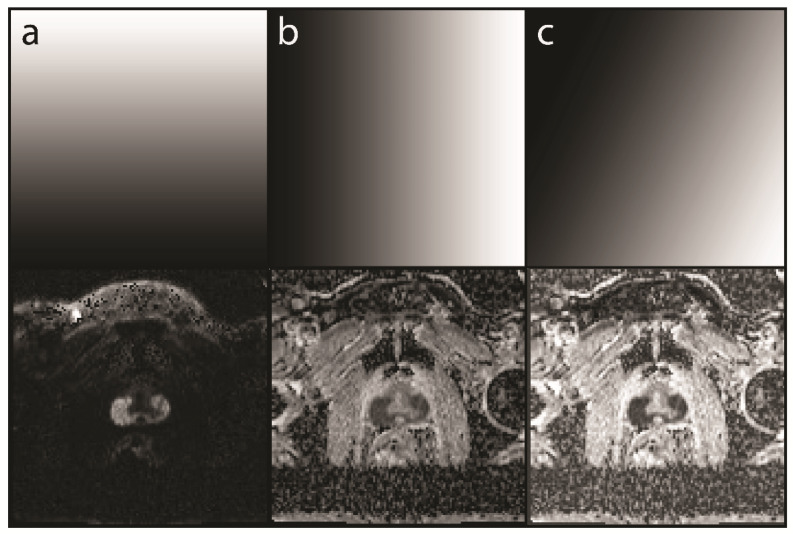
Examples of different readouts from a mcDI dataset created from a high b-value DWI (hbDWI), the ADC, and an intensity map. The selected map is shown in the top row and the resulting mcDI in the bottom row. (a) A readout with a strict vertical gradient yields the original DWI. The resulting image contains no ADC information; (b) In contrast to (a), a strict horizontal gradient yields the original ADC image; (c) A tilted gradient yields the mcDI containing both the DWI and ADC information with an even lower signal in the large tumor in the peripheral zone of the prostate and a lighter background.
